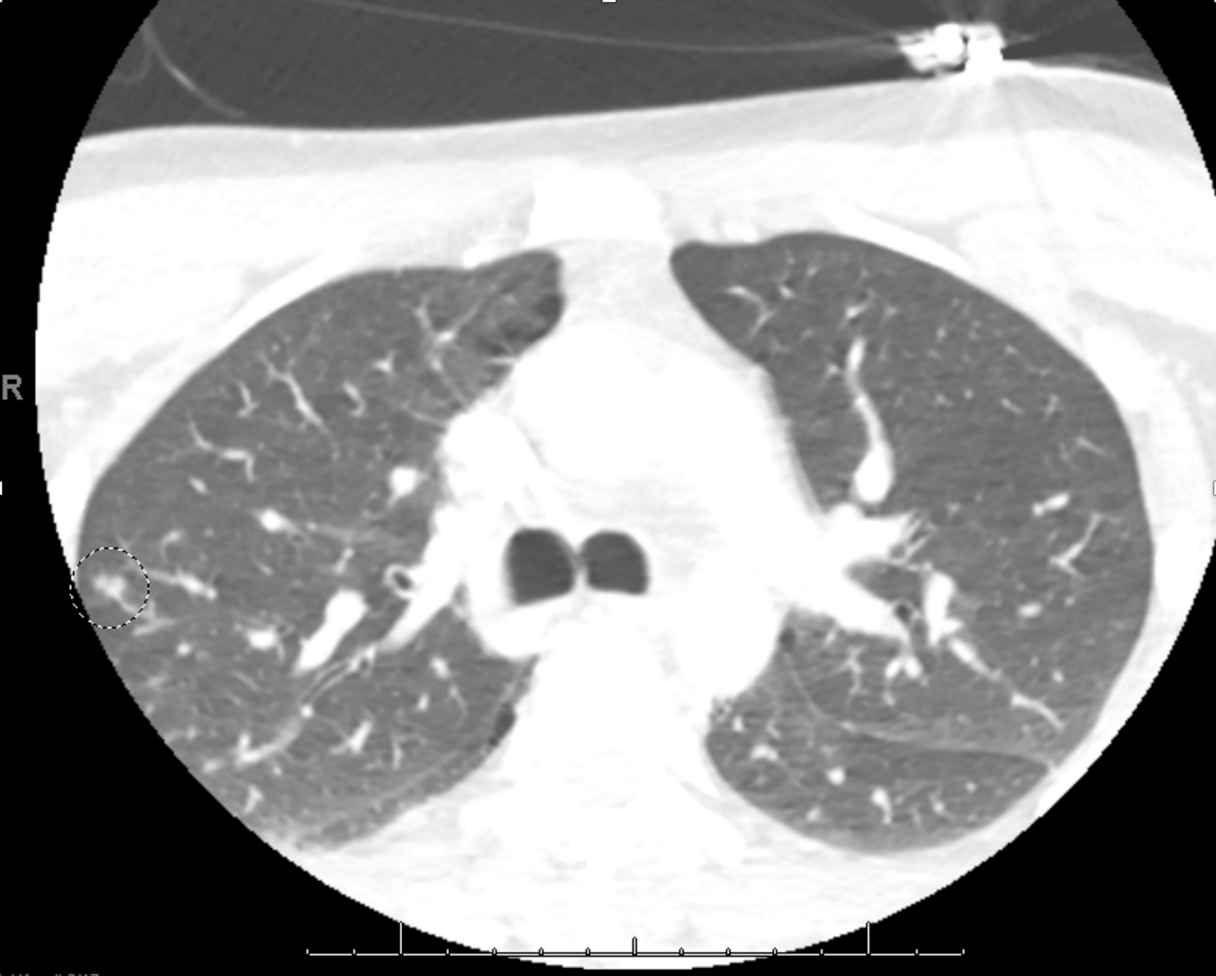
Initial contrast-enhanced CT axial image showing a small, well-defined nodule in the right lung (circled area) representing a septic embolus.
